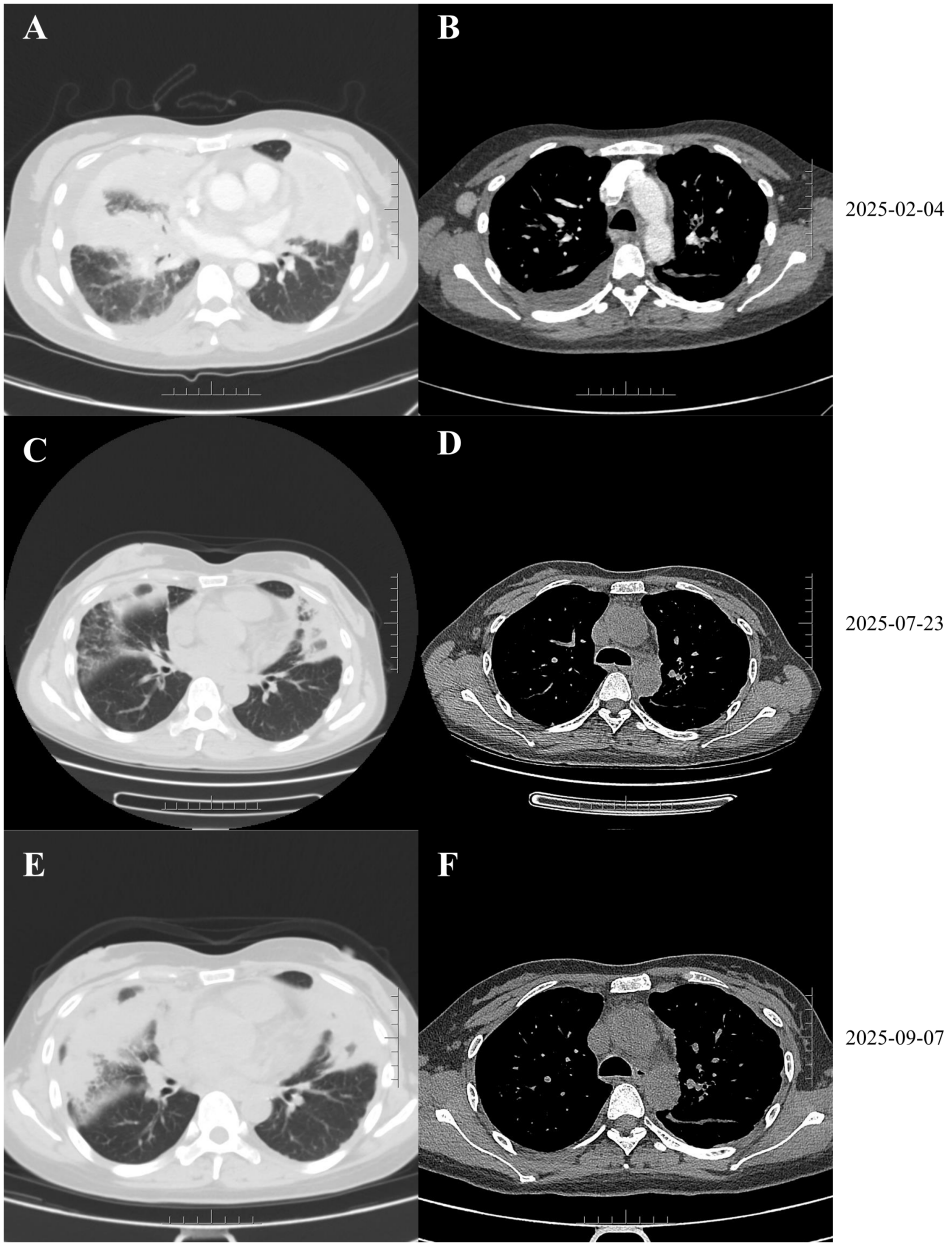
(A, B) The mass in the lingular segment of the left upper lobe further enlarged to 8.4 × 4.4 cm. This was accompanied by multiple nodular, patchy, and cord-like high-density opacities in both lungs and enlarged right axillary lymph nodes. (C–F) Follow-up CT scans obtained during treatment with ivonescimab demonstrated a reduction in the size of both the pulmonary lesions and lymph nodes compared to pre-treatment imaging.
